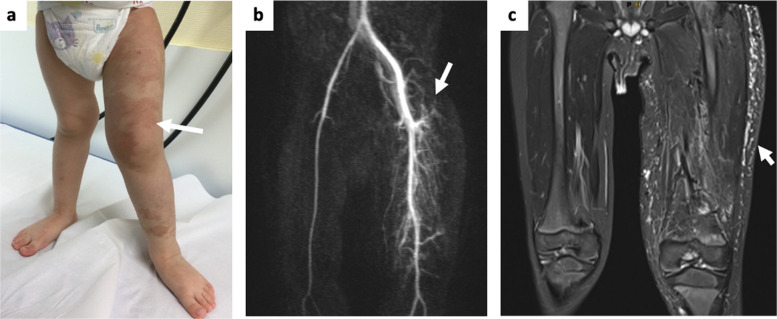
(Pat. No. 57). 2-year-old male patient presenting with a Parkes Weber syndrome-like phenotype of the left leg and somatic *BRAF* pathogenic variant. a Clinical presentation of the affected left leg with soft tissue hypertrophy of the thigh and confluent capillary malformations (arrow) with noticeable hyperthermia; furthermore, leg lengths discrepancy (left > right, 2 cm) was observed. b Time-resolved angiography with interleaved stochastic trajectories (TWIST) magnetic resonance angiography (MRA) image showing fine-fistulous AVM-components of the left thigh (arrow). c. T2-weighted turbo spin echo (TSE) MR image presenting the extent of the AVM components and accompanied soft tissue hypertrophy and edema (arrow)
